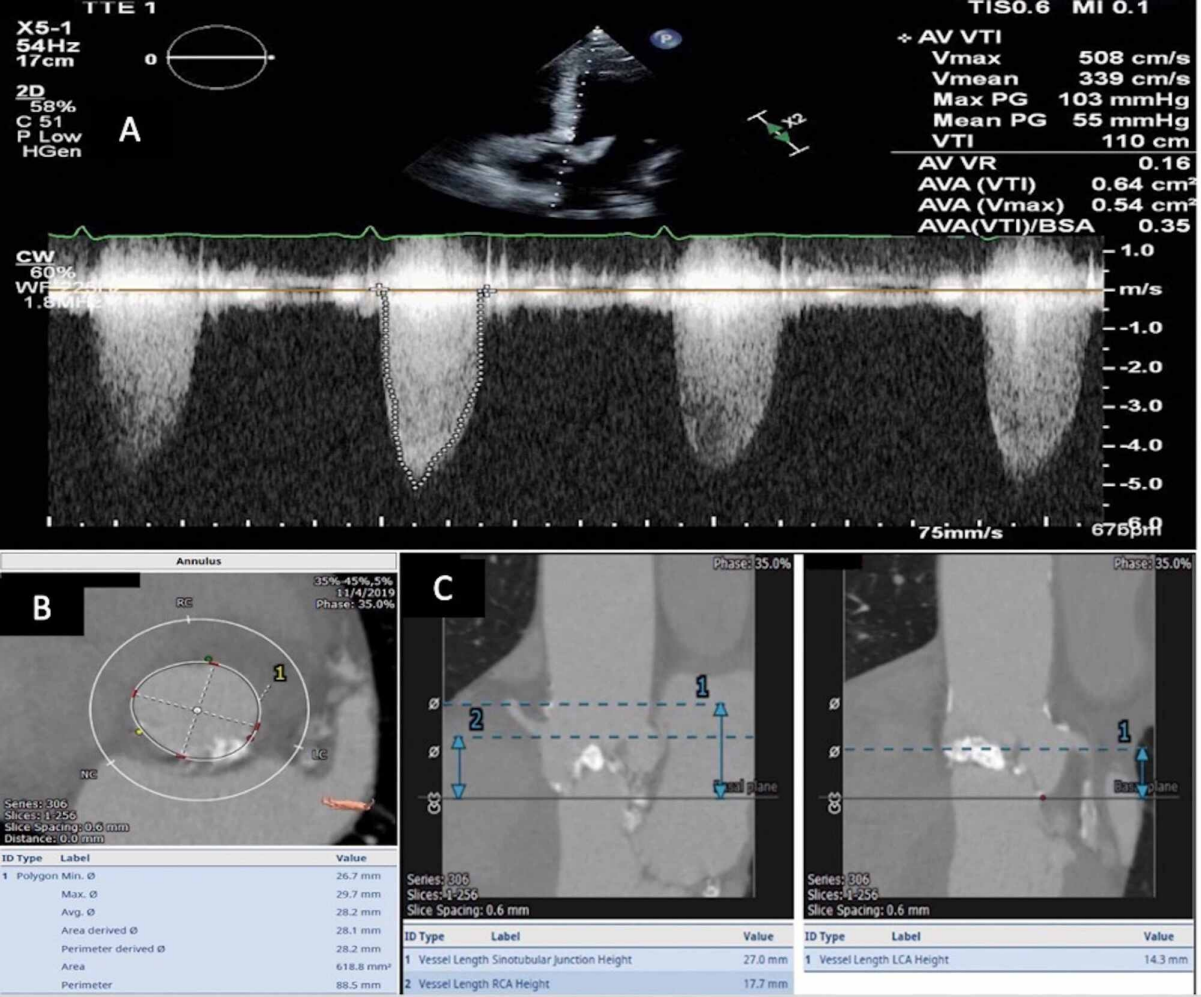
A: continuous wave doppler across the aortic valve; B: aortic annular dimensions; C: right and left coronary ostial heights from the aortic annulus
